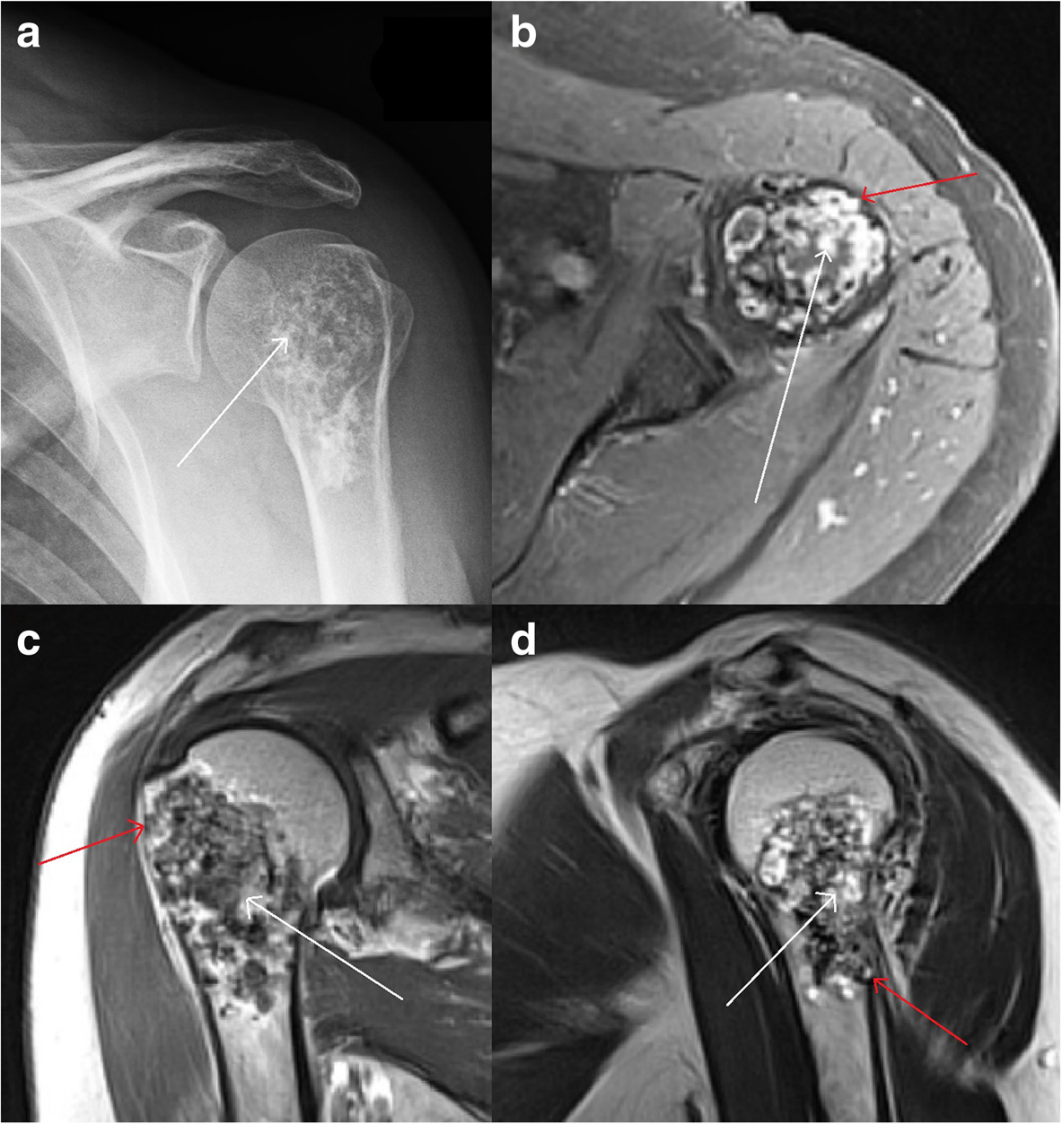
Conservative treatment at the proximal humerus Typical chondroid matrix (white arrows) is visible in ap X-ray (a) and axial (b), coronal (c), and sagittal (d) T1 dotarem contrast-enhanced MRI. Local pain, size and radiological signs of aggressiveness with endosteal scalloping (red arrows) caused presentation and follow-up in our orthopaedic oncology department. The patient declined surgical excision, and was regularly followed-up with MRI and x-ray to rule out significant tumor growth or increase in endosteal scalloping.
